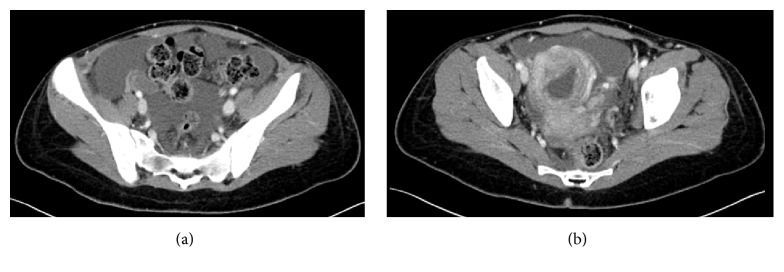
CT pelvis showing haematoma extending to pelvis. Gravid uterus is visible.
